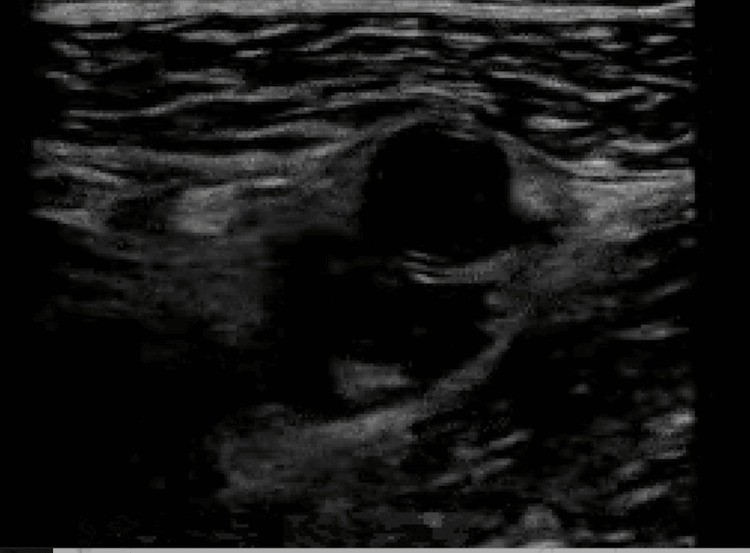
Ultrasound of the right lower extremity demonstrating an acute partially occlusive DVT involving the right mid femoral vein DVT: deep vein thrombosis
